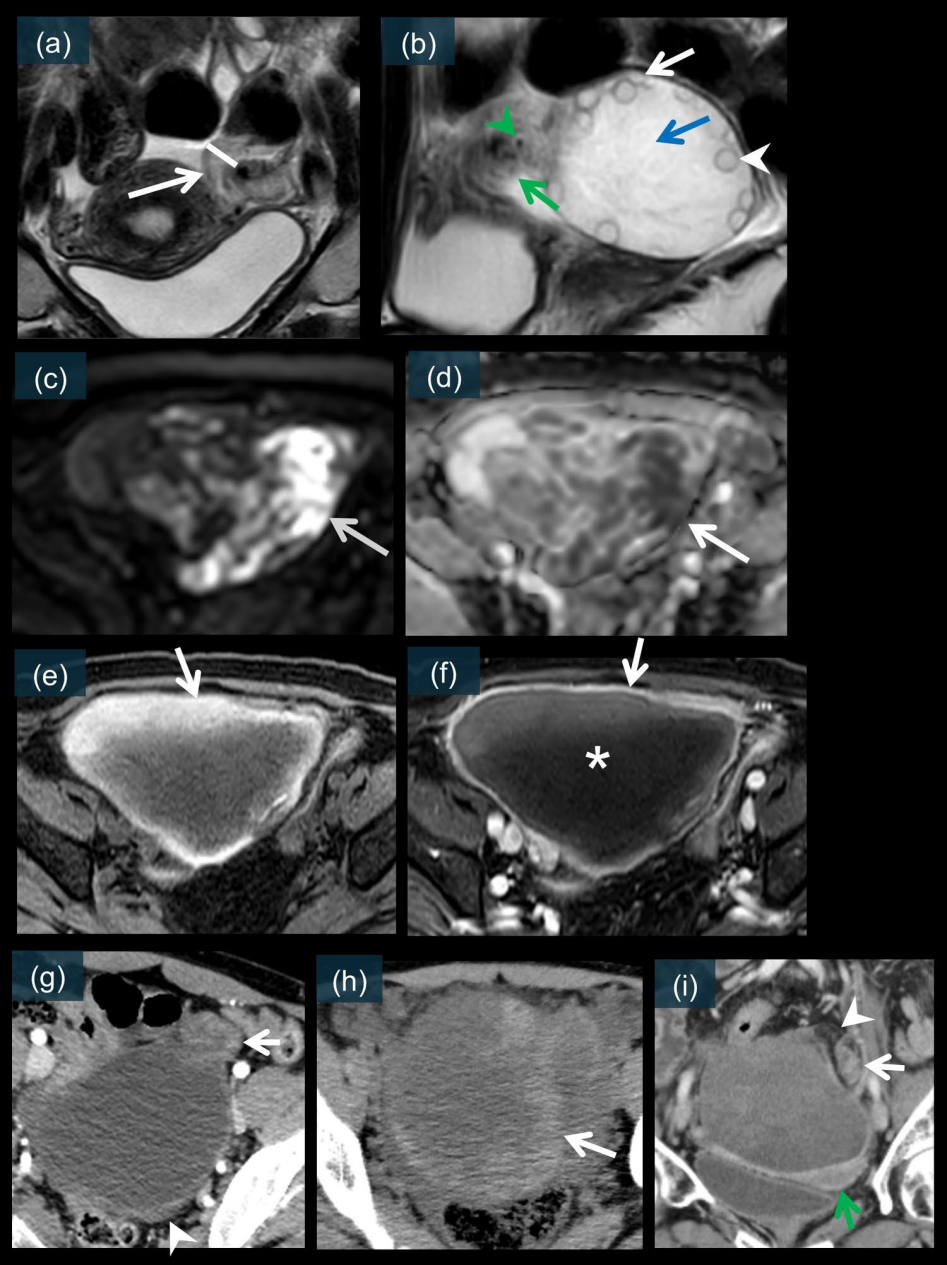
Key diagnostic signs of adnexal torsion on cross-sectional imaging. a Axial T2-weighted image showing fallopian tube thickening exceeding 10 mm (white arrow). b Sagittal T2-weighted image demonstrating the “string of pearls” sign with peripherally displaced follicles (white arrow), a perifollicular T2 hypointense rim (white arrowhead), and stromal edema (blue arrow) in massive ovarian edema. A target-like appearance of the twisted vascular pedicle is also seen (green arrow), characterized by a peripheral high T2 signal with a central flow void (green arrowhead). c Axial diffusion-weighted image (DWI) showing high signal intensity (white arrow) in the ovarian fibroma. d Apparent diffusion coefficient (ADC) map showing corresponding low values (white arrow), confirming restricted diffusion. e Axial T1-weighted image revealing peripheral high signal intensity (white arrow) areas indicating hemorrhage. f Axial contrast-enhanced T1-weighted image showing absent central enhancement (white asterisk) with preserved peripheral enhancement (white arrow). g Sagittal T2-weighted image demonstrating the "whirlpool sign"—a twisted vascular pedicle between the uterus and the affected adnexa (white arrow)—and stromal wall thickening (white arrowhead). h Unenhanced CT image showing hemorrhagic infarction with high-attenuation areas within the enlarged ovary (white arrow). i Contrast-enhanced CT image demonstrating uterine deviation toward the affected side (green arrow), surrounding fat stranding (white arrowhead), and a twisted pedicle (white arrow)
